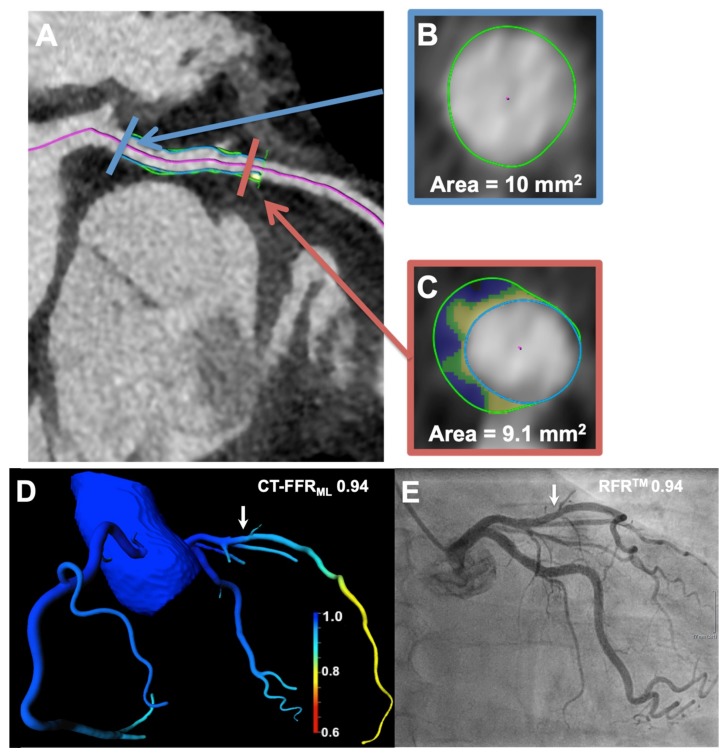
(A) The acquired cCTA shows non-relevant stenosis of the LAD with color-coded automated lesion quantification by the plaque tool. The mixed plaque in the LAD demonstrated a remodeling index of 0.91 and was calculated as (C) the target-lesion cross-sectional area (marked in orange). (B) divided by proximal reference-cross-sectional area (marked blue). (D) A 3-dimensional color-coded reconstruction calculated CT-FFR_ML_ value of 0.94 (CT-FFR_ML_ cut-off value ≤ 0.80) is presented (arrow). (E) This stenosis (arrow) was visualized by ICA and was invasively measured using RFR^TM^ (0.94; RFR^TM^ cut-off value ≤ 0.89), indicating no hemodynamic relevance. cCTA = coronary computed tomography angiography; CT-FFR_ML_ = fractional flow reserve derived from coronary computed tomography angiography based on machine learning algorithm; ICA = invasive coronary angiography; LAD = left anterior descending artery; RFR^TM^ = resting full-cycle ratio.
